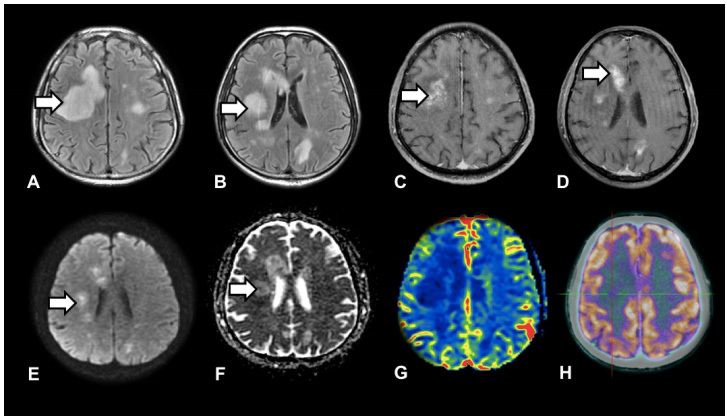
Magnetic resonance imaging findings of intravascular lymphoma. (A and B) Fluid-attenuated inversion recovery axial images showed diffuse multiple hyperintensities of the cerebral white matter. (C and D) The perivascular curvilinear enhancement on T1-weighted imaging with gadolinium expanded markedly. (E and F) Diffusion-weighted imaging and apparent diffusion coefficient map showed restricted diffusion. (G and H) Perfusion magnetic resonance imaging (relative cerebral blood volume) and positron emission tomography - computed tomography were practically unremarkable.
